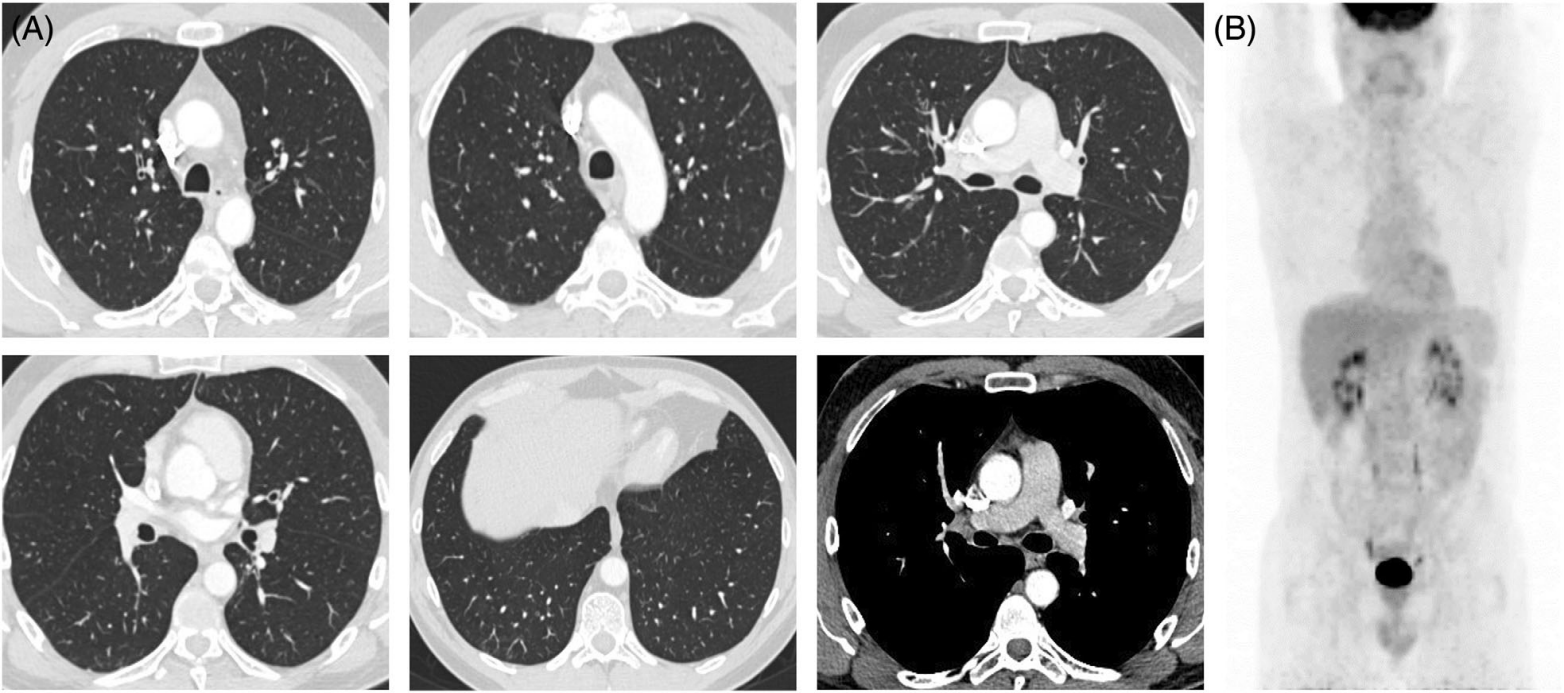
Chest CT‐scan (A) and body PET‐scan (B) after rituximab: Full regression of lung lesions
